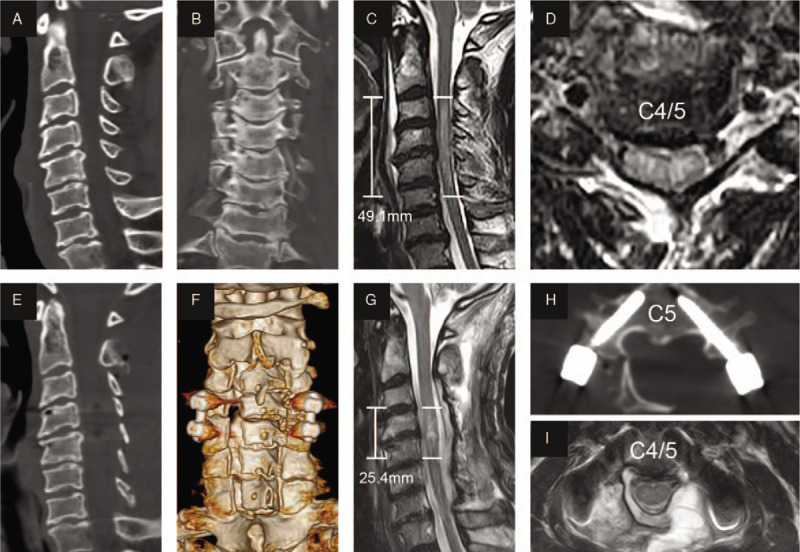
Representational preoperative (A–D) and postoperative (E–I) images of a 60-year-old male patient who sustained a spinal cord injury with posterior ligament injury. His AMS was 24 and AIS grade C. Midsagittal subaxial CT (A) indicated cervical spinal stenosis (CSS) from C3 to C6, and MRI (C) SCI with an intramedullary lesion length (IMLL) of 49.1 mm. The subarachnoid space (SAS) was absent at C4 and C5 (C and D). Laminoplasty of C3–7 and pedicle screw fixation of C4–5 were performed in this patient 13 h after trauma. Postoperative CT (E, F, and H) showed significant enlargement of the osseous spinal canal and good screw positioning. Postoperative MRI showed an IMLL of 25.4 mm (G) and successful decompression (G and H) indicated by the presence of an open anterior and posterior SAS 11 days after trauma. Fourteen days after injury, the patient recovered paralysis with an AMS of 52 and AIS grade D. The patient had moderate hyponatremia and no hypotension or tracheotomy during hospitalization.
